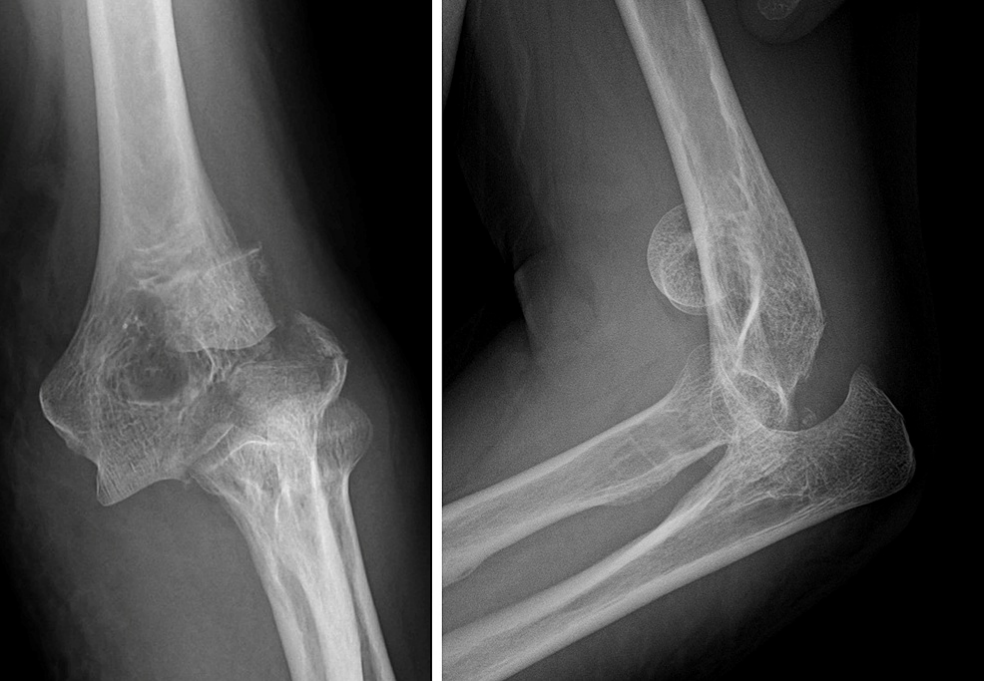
A preoperative radiograph of case 2 showing a fracture from the lateral distal humerus to the trochlea and dislocation to the radial side, with the double arc sign evident on the lateral view.
